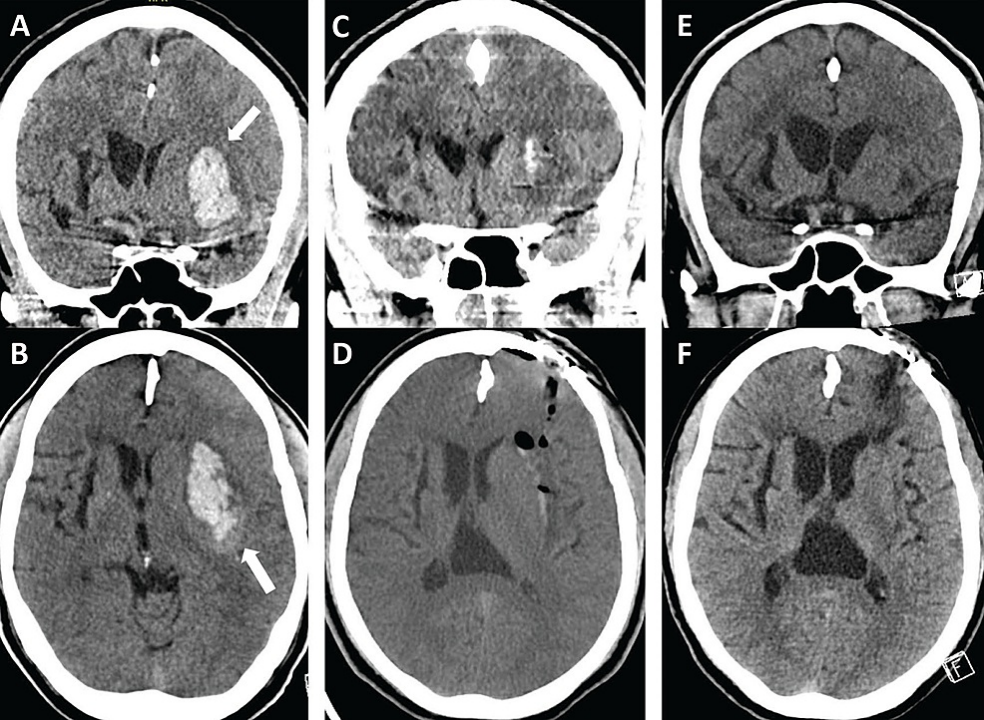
Case 1 - second admission pre- and postprocedure and follow-up imaging. Preprocedure second admission non-contrast coronal (A) and axial (B) head computed tomography (CT) demonstrate a 29.8 mL left basal ganglia hemorrhage (arrow).  Postprocedure coronal (C) and axial (D) non-contrast CT demonstrated near-complete evacuation, with a 95.0% evacuation rate and remaining hematoma measuring 1.5 mL. Coronal (E) and axial (F) follow-up imaging more than three months post hemorrhage show resolution and expected encephalomalacia.
